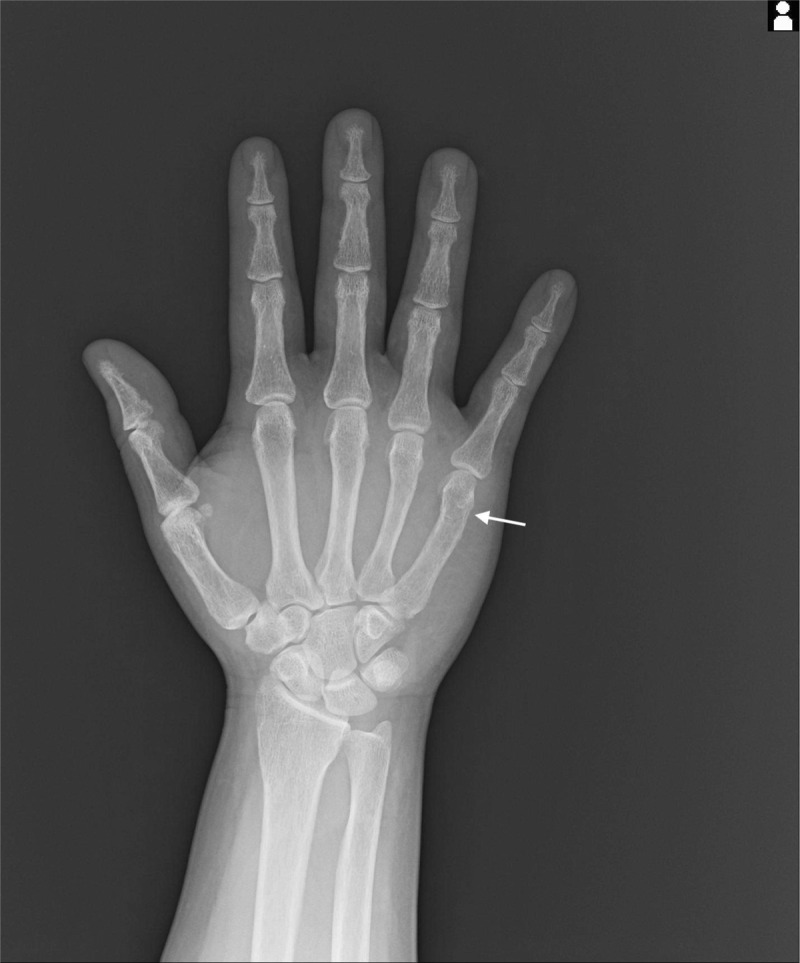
An x-ray showing the metacarpal bone fracture at right fifth bone of the patient on the day of the injury (arrow).
